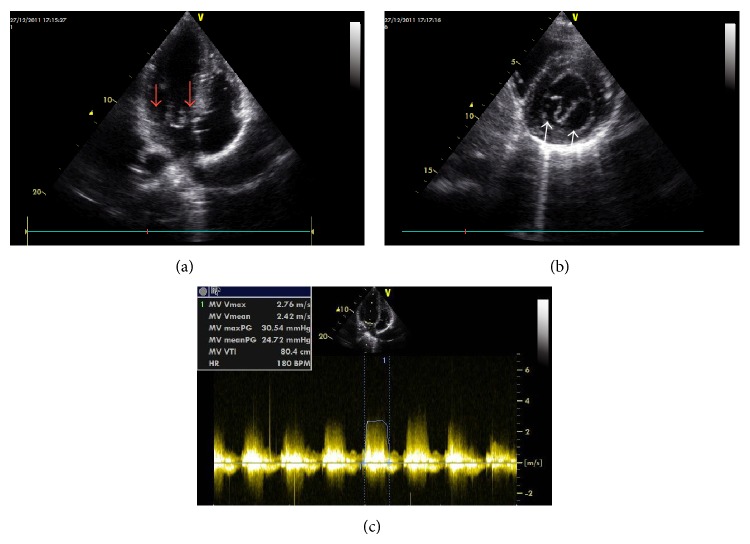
(a) Apical four-chamber view and (b) parasternal short axis view showing double orifice mitral valve. (c) Continuous wave Doppler tracings showing severe gradient over mitral valve.
